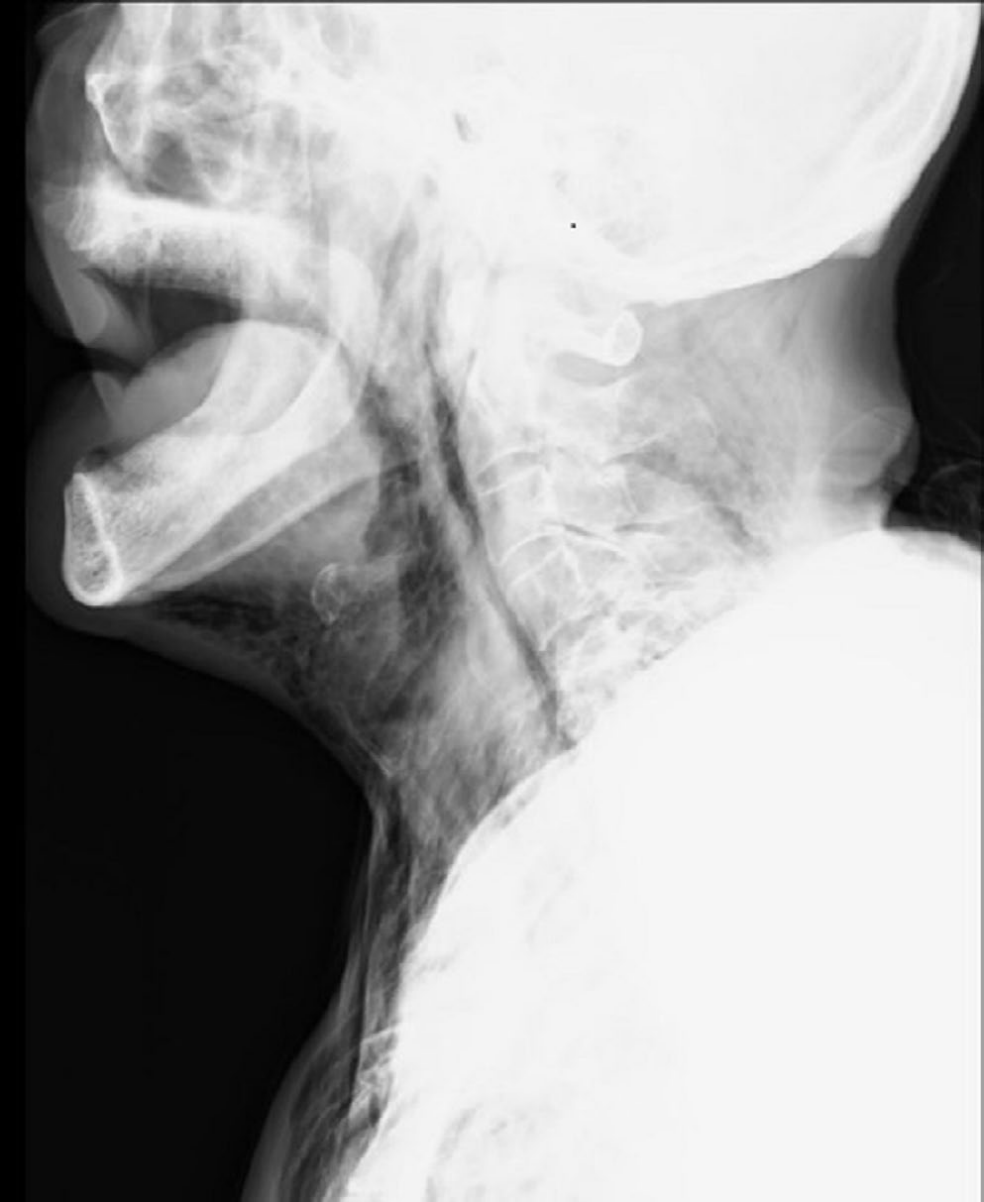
Radiograph of the neck showing pneumomediastinum, extending to the laryngopharynx, and air in the retropharyngeal space
